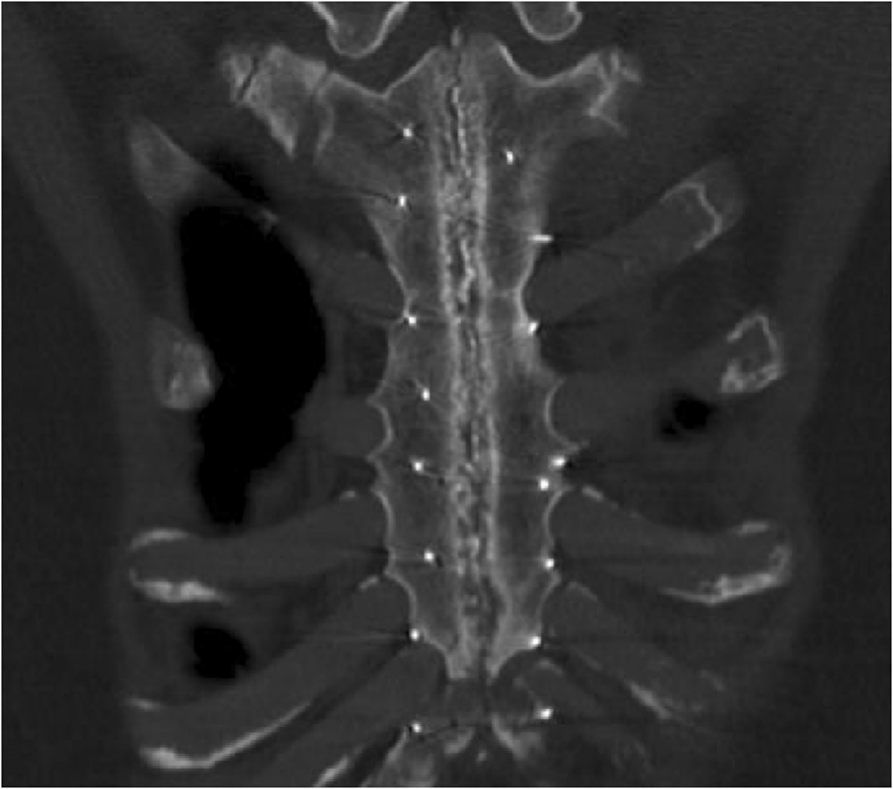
Computed tomography of chest showing sternal union in a patient who had adhesive-enhanced closure.
